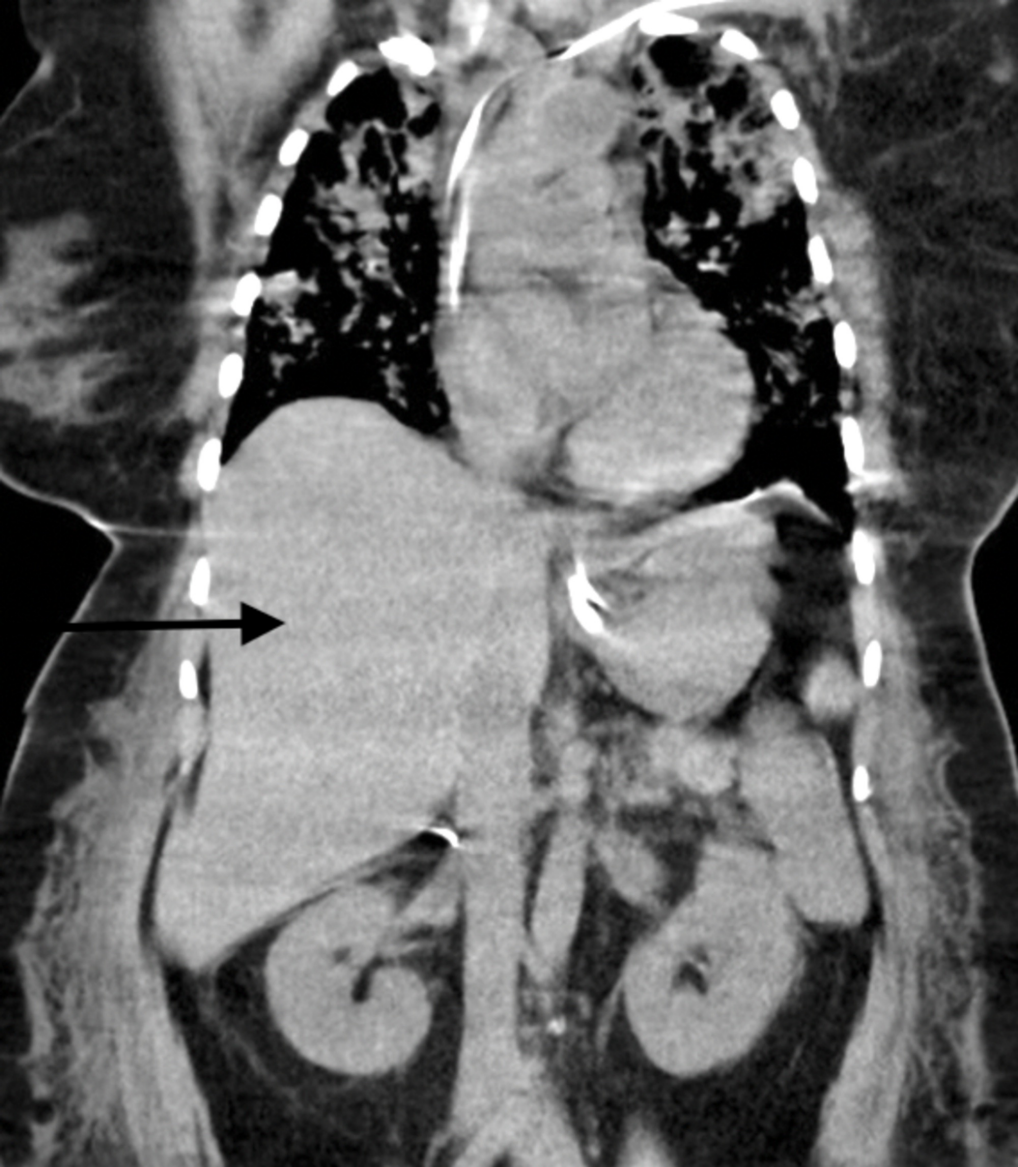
Non-contrast CT scan showing normal appearance of liver on the 8th day of hospitalization
